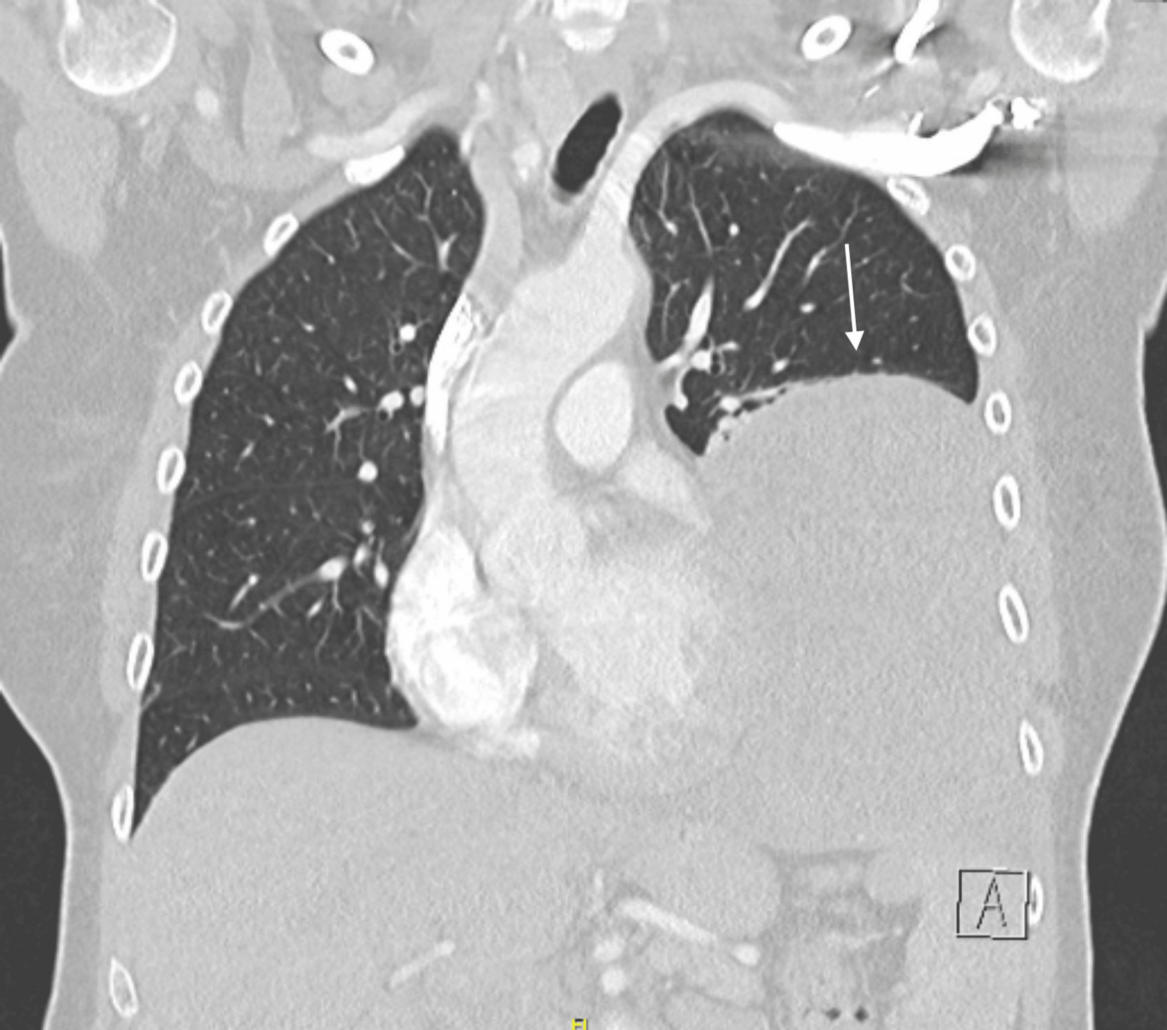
CT of the thorax showed a mass in the lower lobe of the left lung. Coronal plane, lung window.
